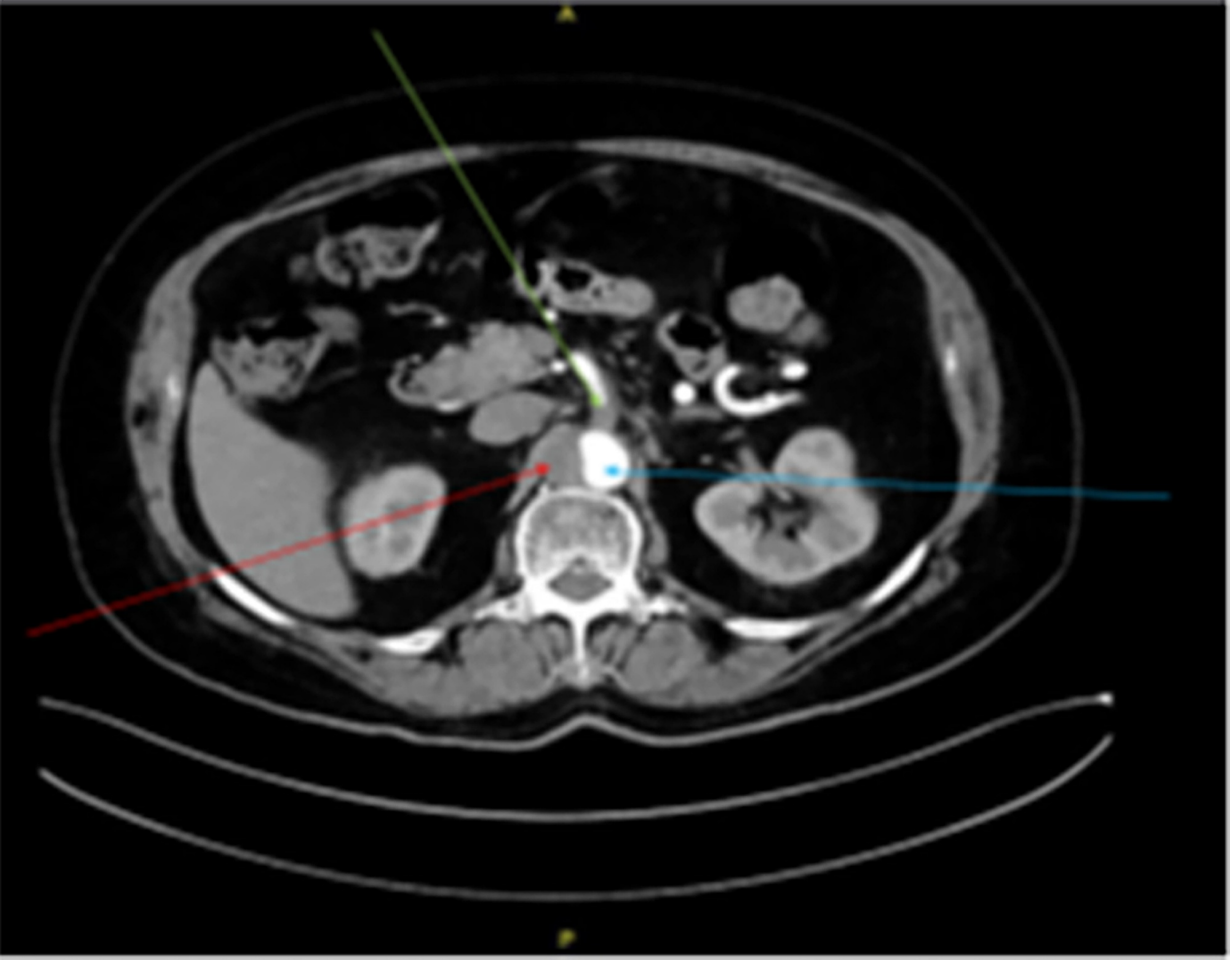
CT with contrast green arrow shows chronic occlusion of SMA, blue arrow shows true lumen of aorta, red arrow shows thrombus in aorta. SMA, superior mesenteric artery
